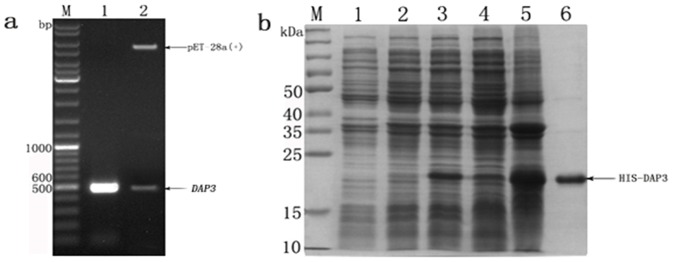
Construction of recombinant plasmid and production of DAP3 recombinant protein. (a) TAE agarose gel electrophoresis of PCR and double digestion analysis. Lane M:Gene Ruler 100 bp plus DNA Ladder; lane 1: PCR with the recombinant plasmid as template; lane 2: Digestion with *Bam*H I and *Hind* III. (b) SDS-PAGE analysis of DAP3 recombinant protein in *E.coli* BL21 cells. Lane M: protein moleculai mass marker; lane 1: *E.coli* BL21 containing expression vector pET28a(+) induced by IPTG; lane 2: *E.coli* BL21 cells containing expression vector pET28a(+)-*DAP*3 without IPTG; lane 3: *E.coli* BL21 cells containing expression vector pET28a(+)-*DAP*3 induced by IPTG; lane 4: Supernatant solution after ultrasonic; lane 5: Precipitation after ultrasonic; lane 6: purified protein by Ni-NTA affinity column.
